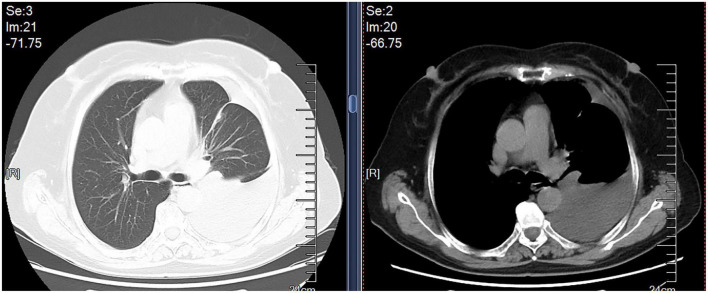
Chest CT scan showed left pleural effusions and linear opacity.
